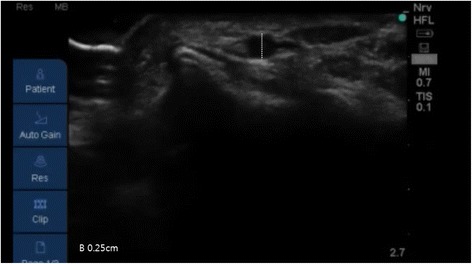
Measurement of the diameter of the radial artery on the frozen image in ultrasound
